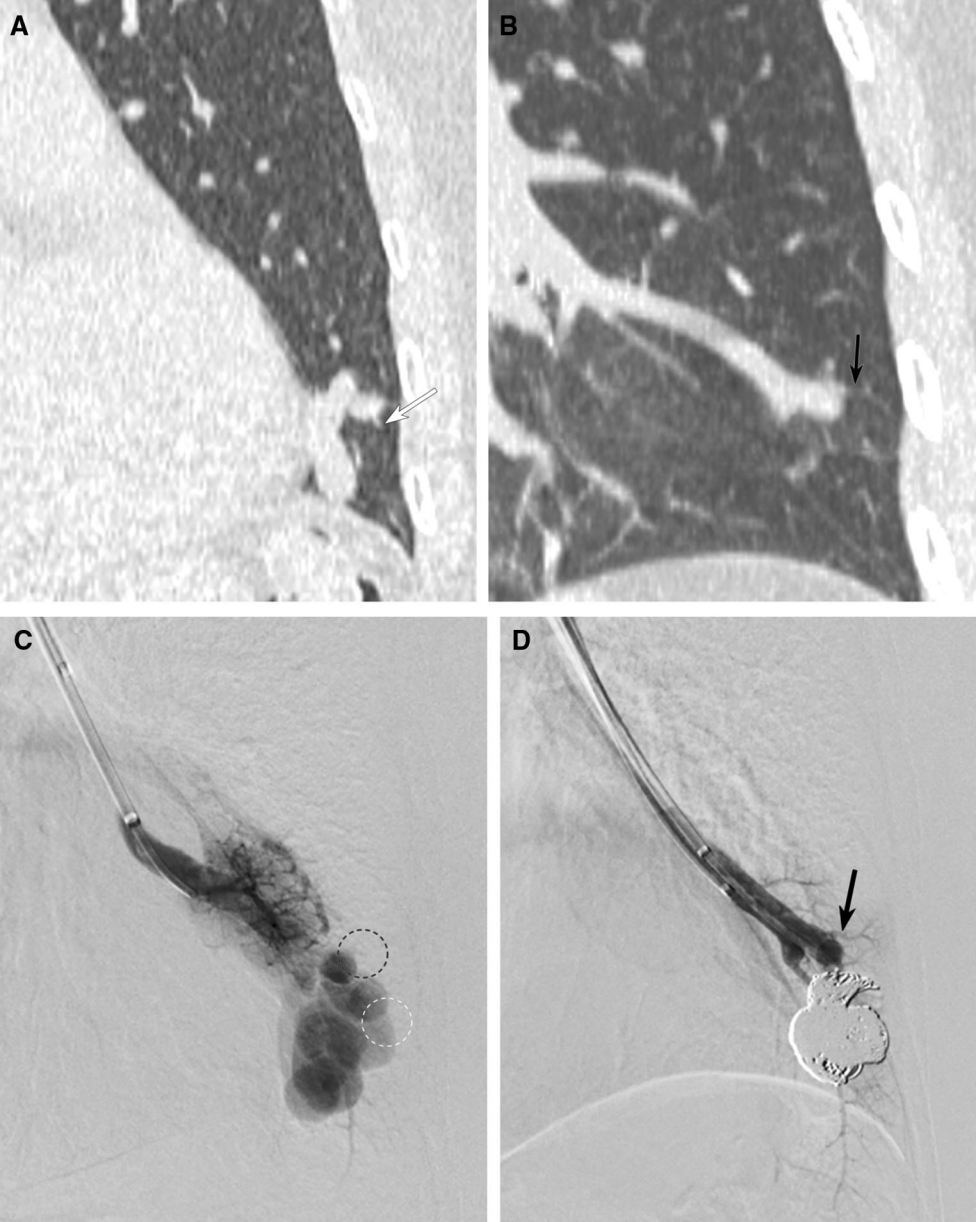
A case of PAVM in which selective PAG failed to demonstrate the last normal branch and proximal branches. A Oblique coronal MPR CT image shows the last normal branch originating from junction (white arrow). B Oblique coronal MPR CT image also shows the proximal branch from feeding artery (black arrow). C Frontal view of selective PAG cannot demonstrate both the last normal branch (circle of white dot-line) and proximal branch (circle of black dot-line) detected on MDCT. D Selective PAG after embolization clearly demonstrates the proximal branch from feeding artery (black arrow) which could not be visualized before embolization (circle of black dot-line in Fig. 4B)
